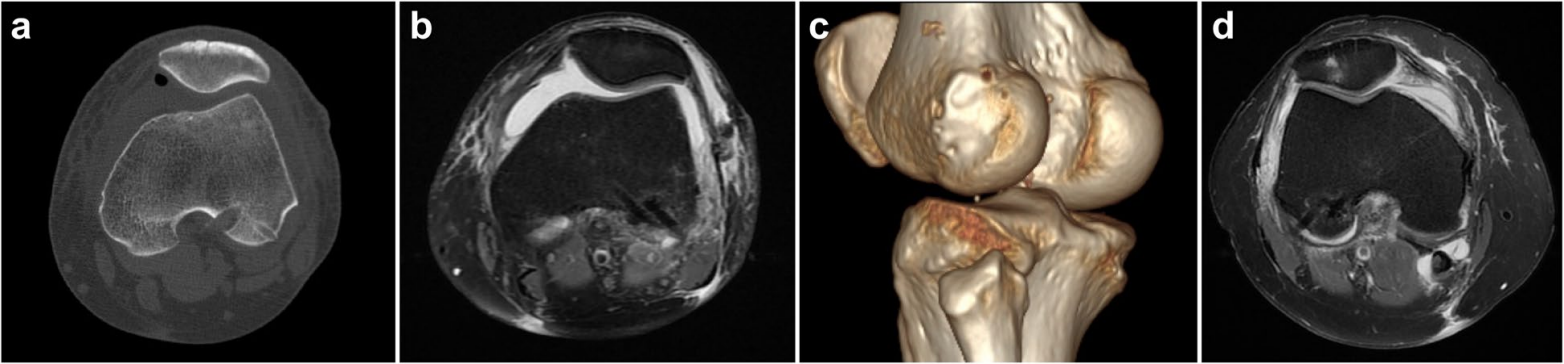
a Cross-pin penetration of the femoral condyle cortex on postoperative CT. b Cross-pin penetration of the femoral condyle cortex on postoperative MRI. c Cross-pin penetration of the femoral condyle cortex on postoperative three-dimensional CT. d Cross-pin penetration of the femoral condyle cortex and injury of the posterolateral joint capsule
